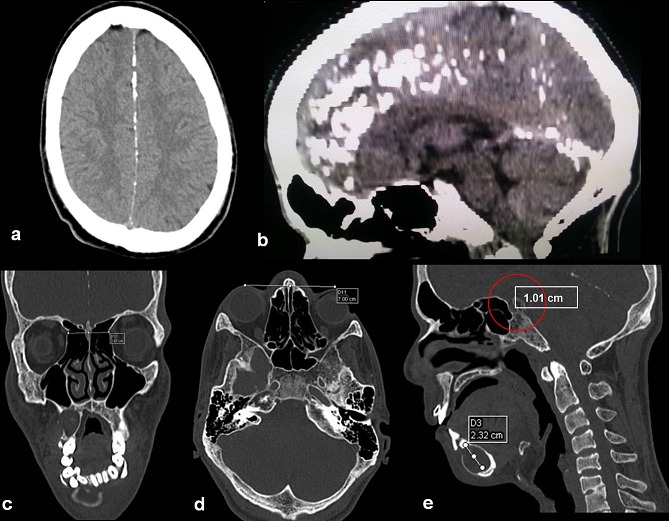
a) CT image showing multiple calcifications along the falx cerebrii; b) tentorium cerebelli; (c,d) linear measurements showing increased inter-canthal, orbit globe distance; e) narrowing of sella turcica
